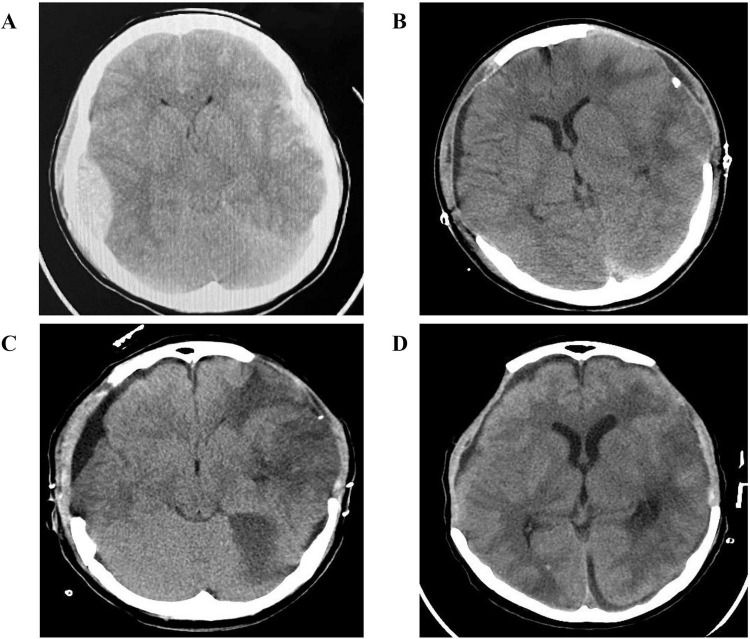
Head CT images of the patient’s on Day 1 (A), Day 11 (B), Day 19 (C), and Day 26 (D).
